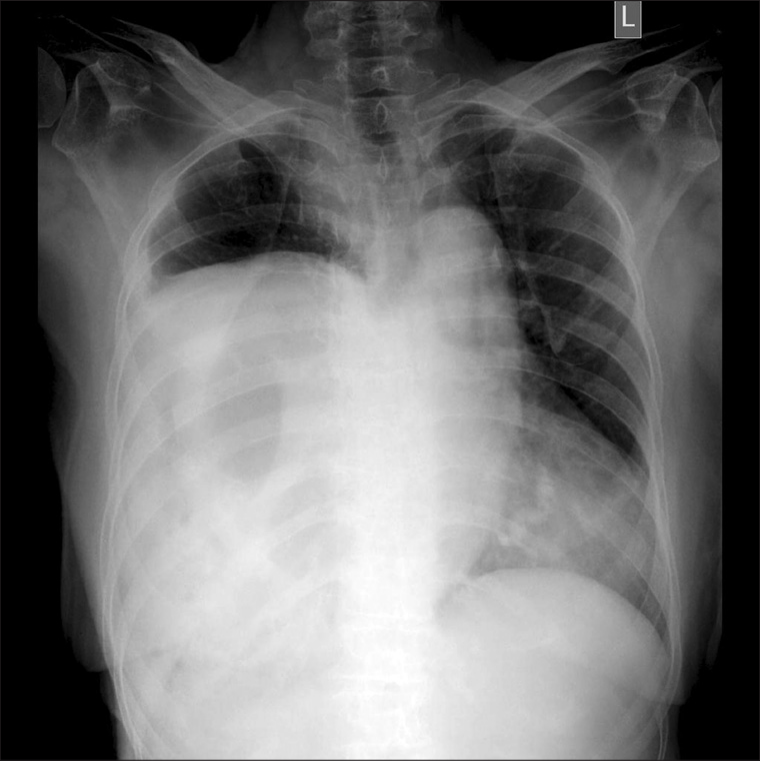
Plain radiograph showed opacification of the entire lower part of the right hemithorax with air lucencies within
